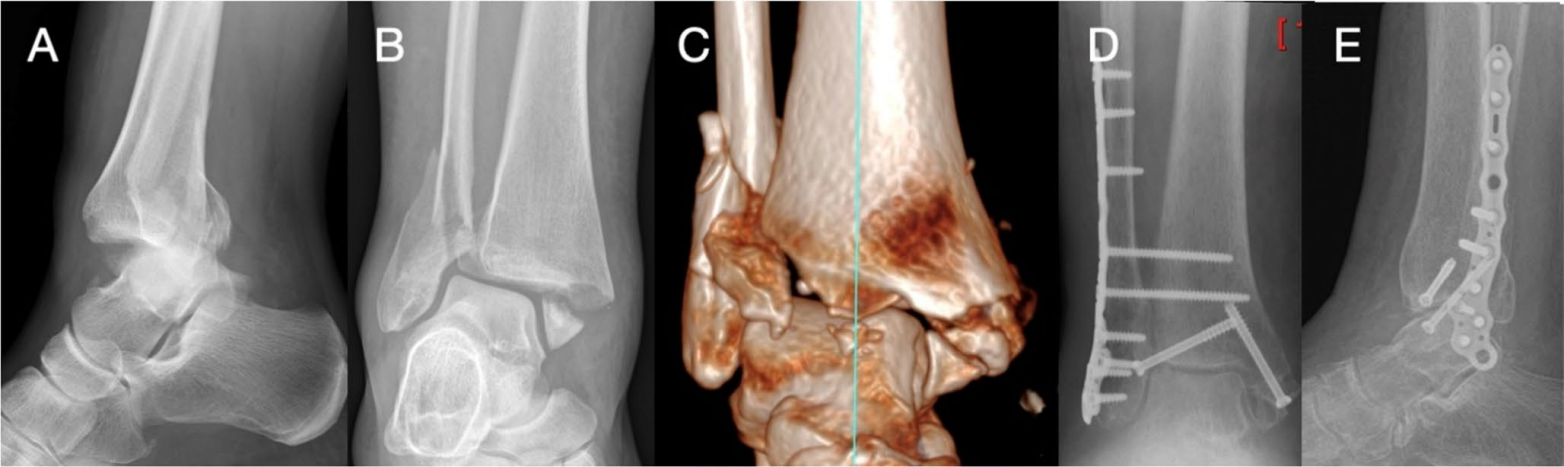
Preoperative lateral (A) and anteroposterior (B) radiographs and 3D CT reconstruction (C) of an unstable ankle. Weight-bearing anteroposterior (D) and lateral (E) radiographs taken at 3 months post extended ORIF fixation using a locking plate and fibular pro tibia fixation
